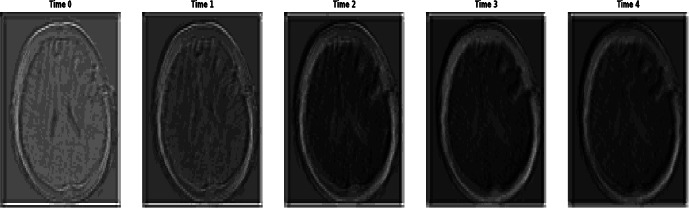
Serial axial CT images show a hyperdense area within the right frontoparietal region, consistent with acute intracerebral hemorrhage or hemorrhagic transformation of infarct at Time 0. The lesion demonstrates well- defined margins with associated surrounding hypo attenuation, indicative of vasogenic edema. Over successive time points (Time 1 to Time 4), there is a gradual reduction in the hyper density, signifying hematoma resorption. The surrounding edema also reduces, and there is no progression of mass effect or ventricular compression. The sukal spaces and adjacent cortical structures appear to retum toward normal. By Time 4, the hematoma appears hypodense, consistent with the chronic phase of hemorrhage resolution. No new lesions, midline shift, or hydrocephalus are seen. Impression: Evolution of a right frontoparietal intracerebral hemorrhage from acute to chronic phase with marked resolution. No imaging evidence of rebleeding, infarct progression, or hydrocephalus. Stable follow-up without new conceming features. Recommendation: Continue clinical monitoring. MRI with GRE/SWI sequences may be beneficial if further evaluation of hemosiderin deposition or residual gliosis is needed.
